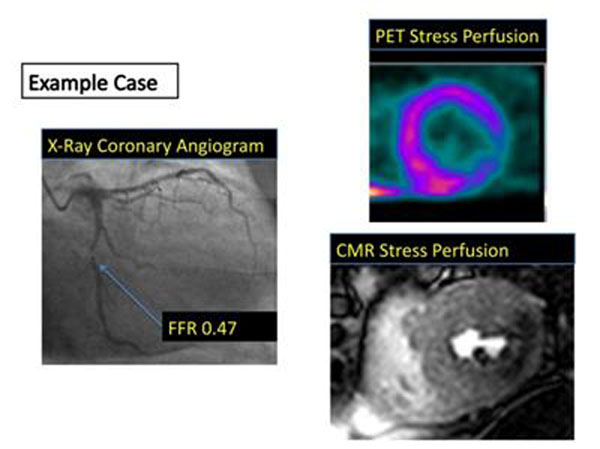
# Comparison of cardiac magnetic resonance imaging and positron emission tomography for the diagnosis and localization of coronary artery disease
